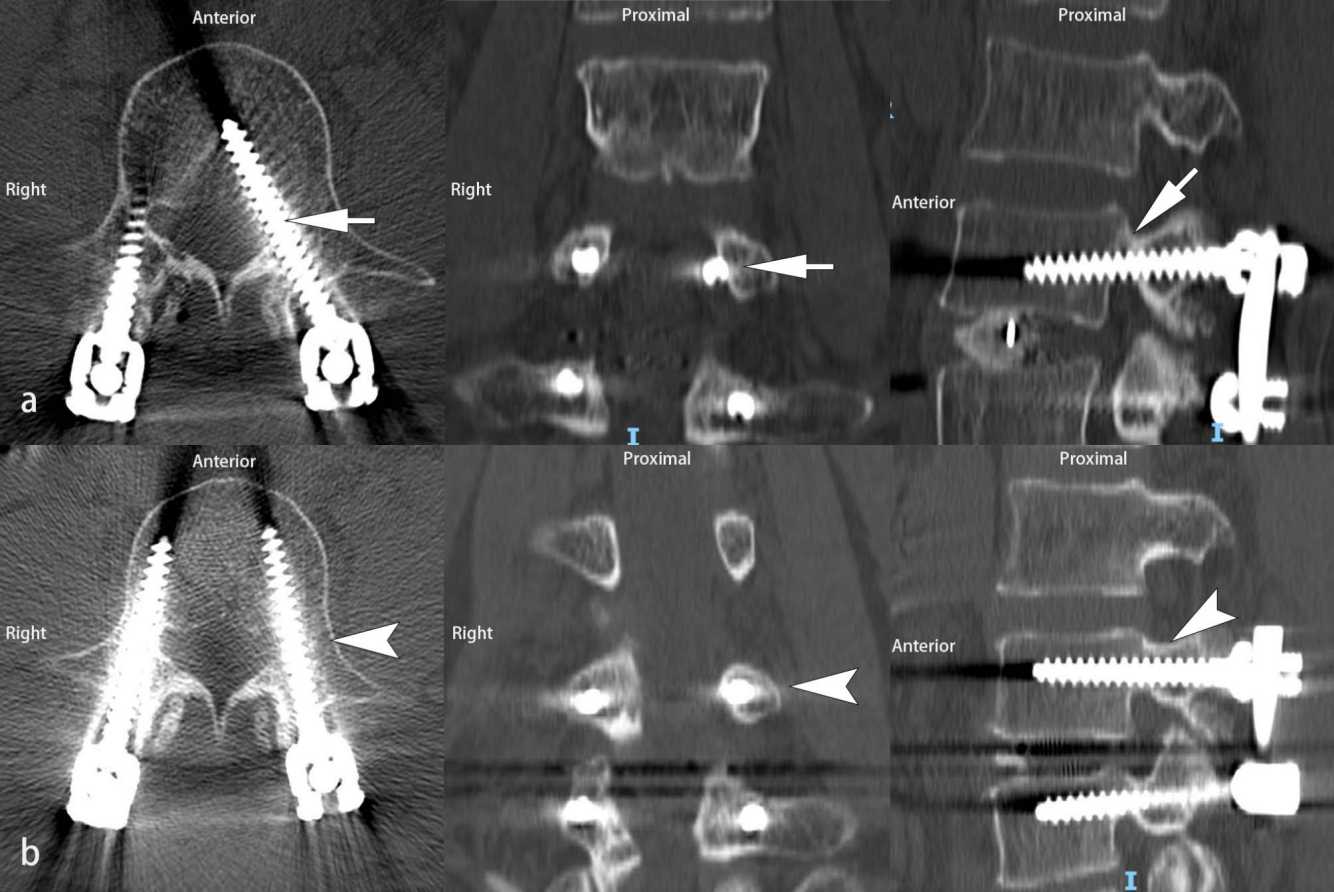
A 65-year-old woman undergoing L4-5 TLIF had severe radiated pain of the left lower extremity with weak muscle strength at postoperative day 1. The postoperative CT scan revealed a major pedicle screw breach (> 6 mm, grade 4) of the left L4 pedicle (a white arrow). Revision surgery was scheduled immediately. The postoperative CT scan showed that the revised screw was inside the pedicle (b, white arrowhead). The patient recovered without consequence
